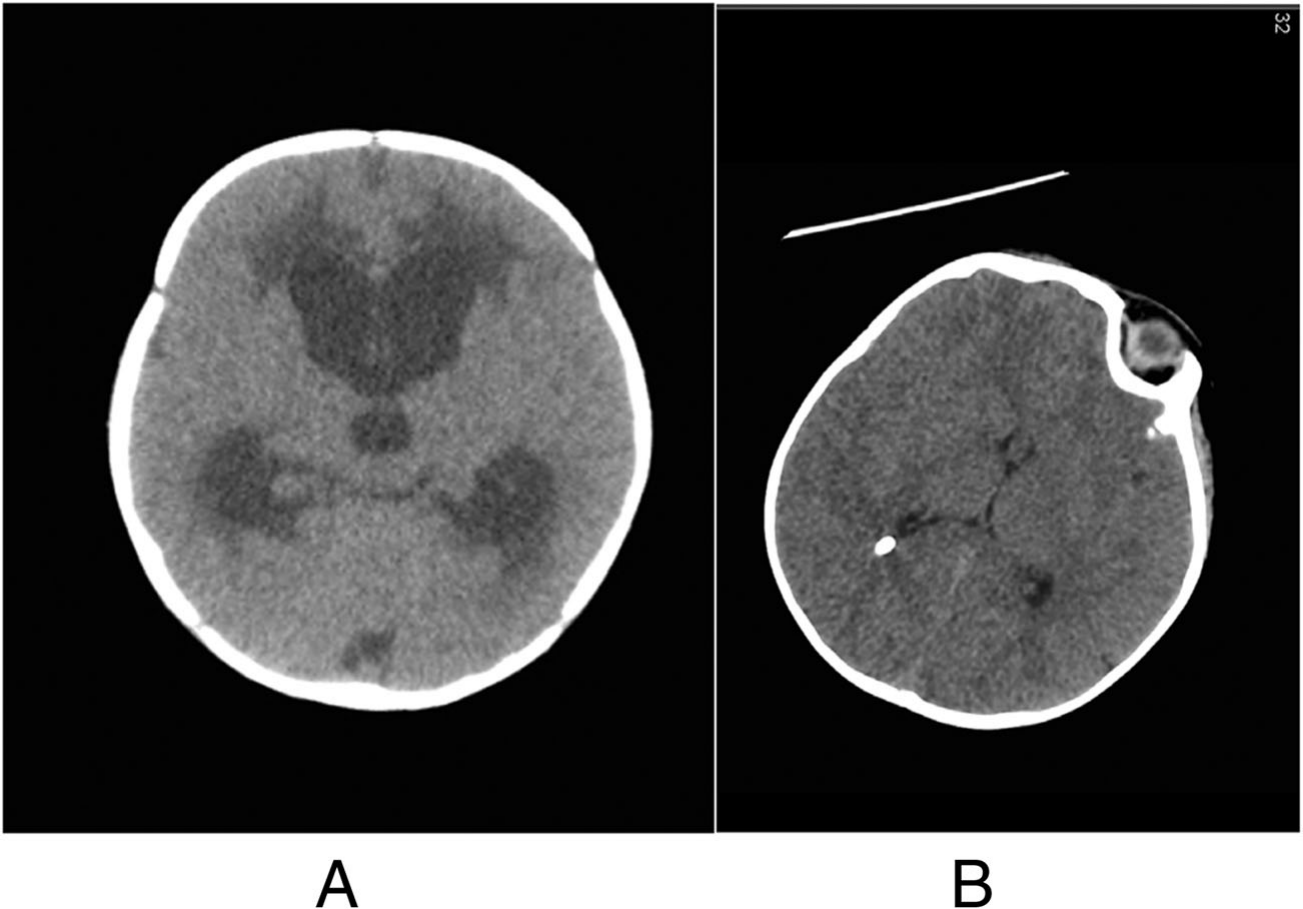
Axial CT. a Communicating hydrocephalous and transependymal oedema. b Decompressed ventricles after shunt placement
